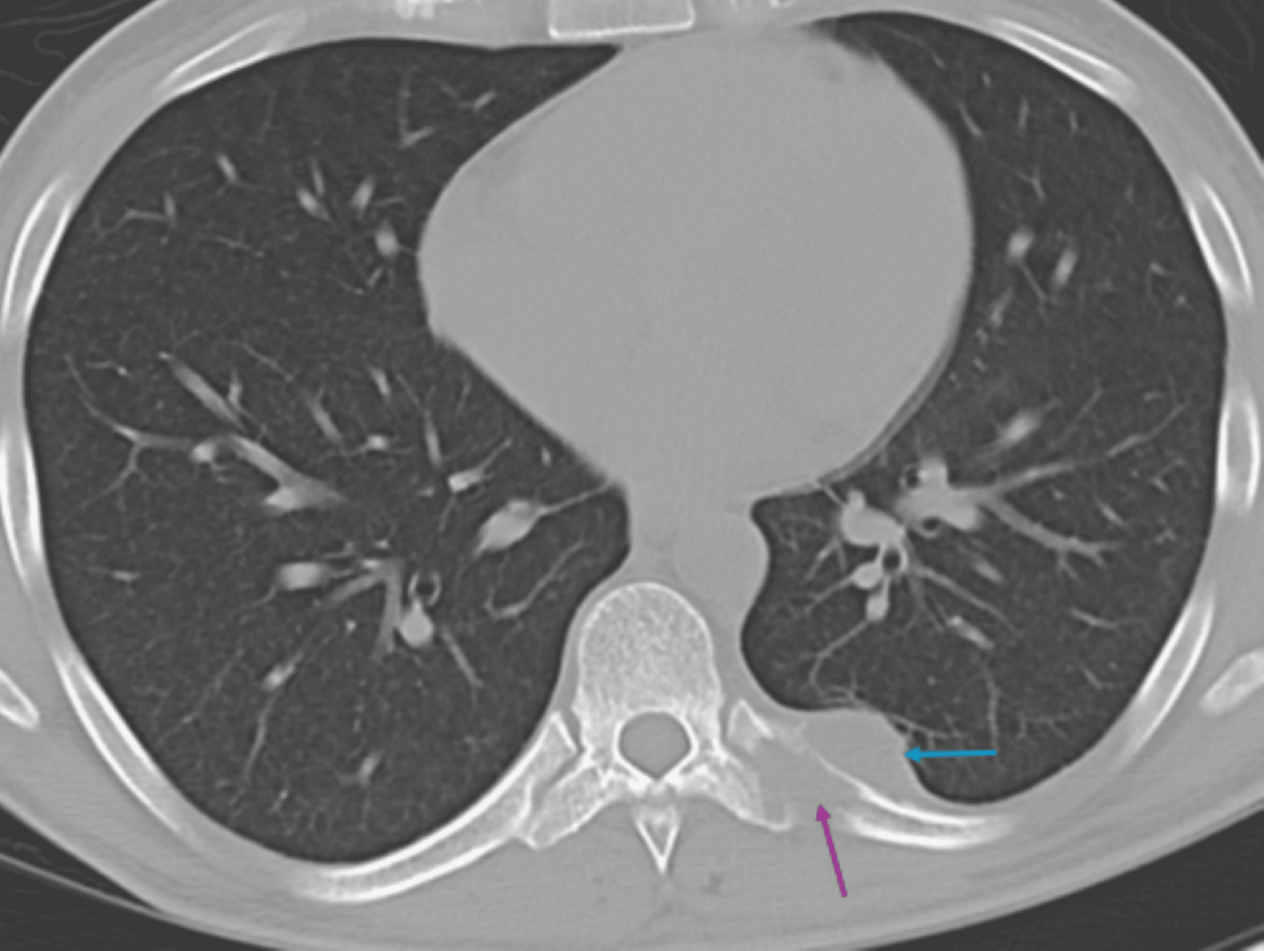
Noncontrast-enhanced axial chest CT demonstrating osteolysis of the posterior left ninth rib and adjacent transverse process consistent with osteomyelitis (purple arrow), with an adjacent pleural-based soft tissue phlegmon (blue arrow) CT: computed tomography
